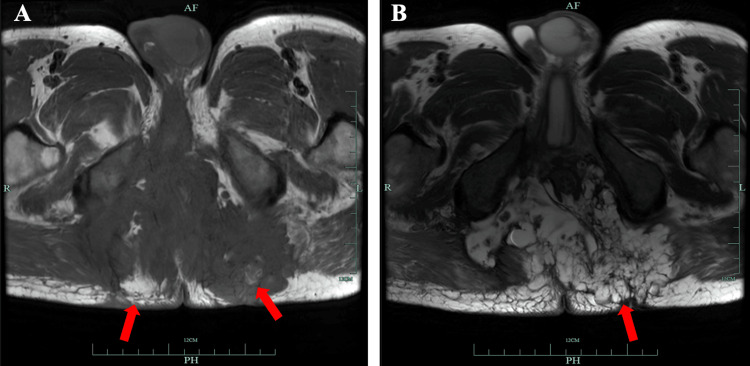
Magnetic resonance imaging of anal fistula. (A) T1-weighted images show patchy, iso-signal lesions around the anal region, with some areas exhibiting patchy high signal intensity (arrows). (B) T2-weighted images show perianal abnormal high-signal lesions. Multiple separated, well-defined, cystic lesions are predominantly seen on the left side of the subcutaneous tissue of the buttocks (arrows).
